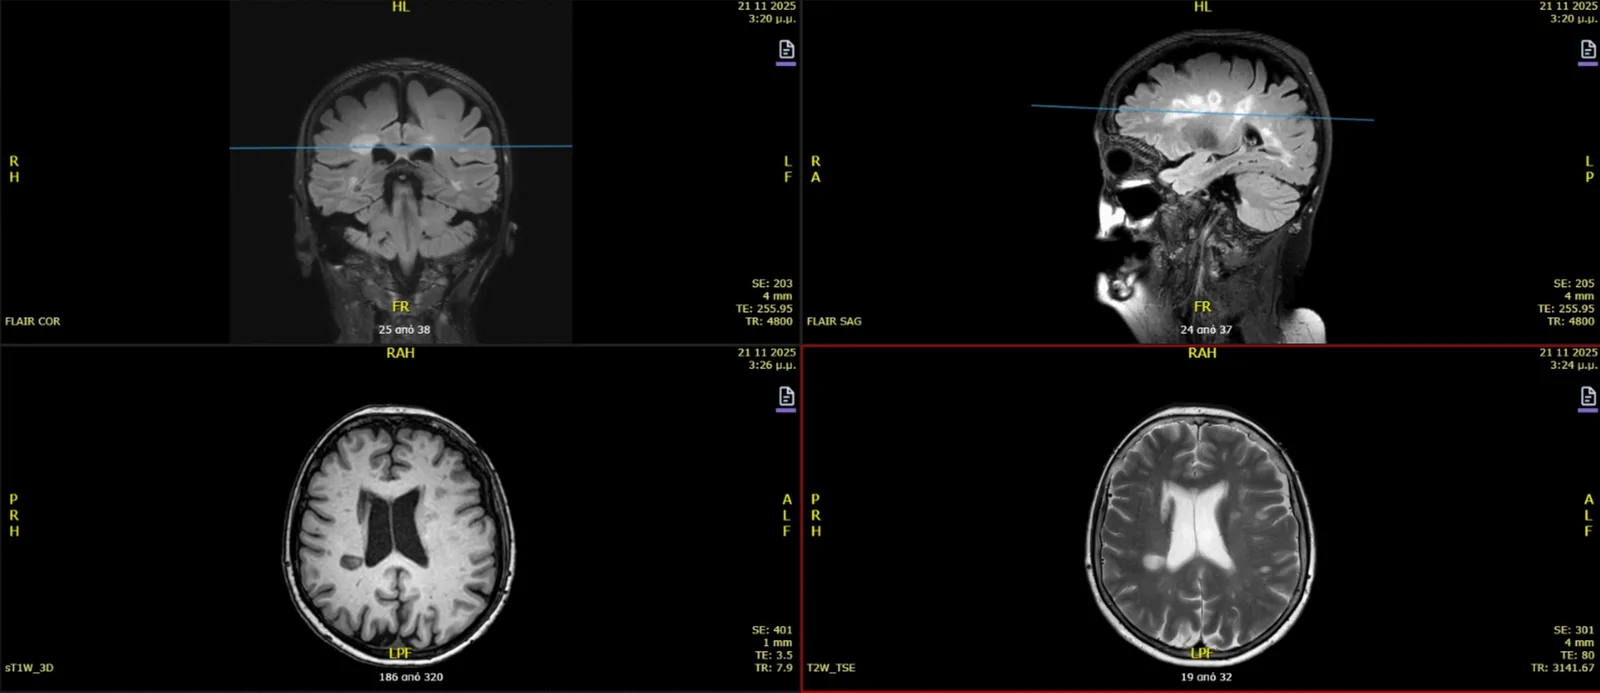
Repeat brain MRI scan of the patient – No new demyelinating lesions are revealed, while a substantial number of the pre-existing ones shows a marked reduction in size. Indicatively, the largest parietal lesion in the right hemisphere has a maximum diameter of 17 mm compared with 25 mm in the previous imaging
